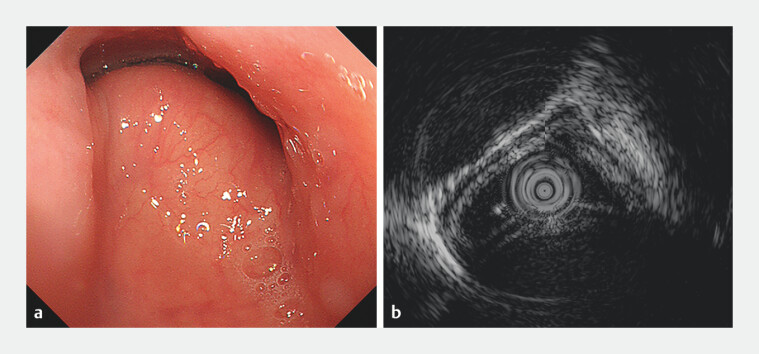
Endoscopic and endosonographic findings of esophageal submucosal protrusions.
